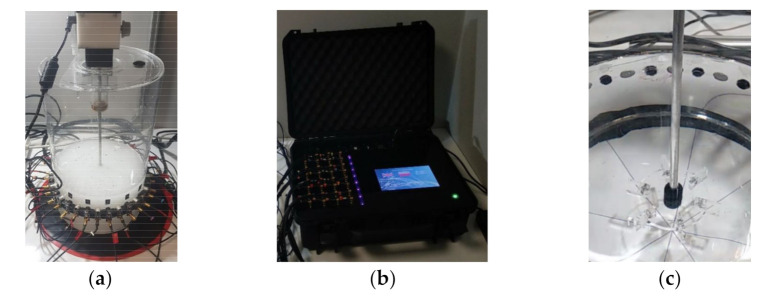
Ultrasound experimental setup. (a) Reactor tank, (b) measurement unit, (c) mixer.
